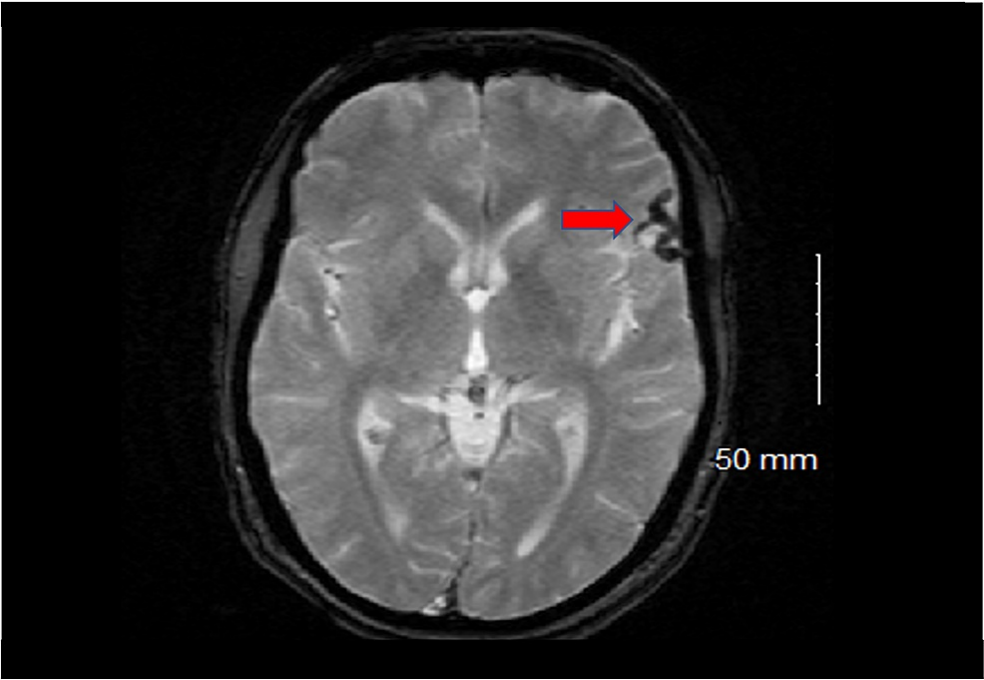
MRI of the brain before surgery A dilated Sylvian vein is visualized adjacent to the cortex of the left frontal lobe (red arrow).
